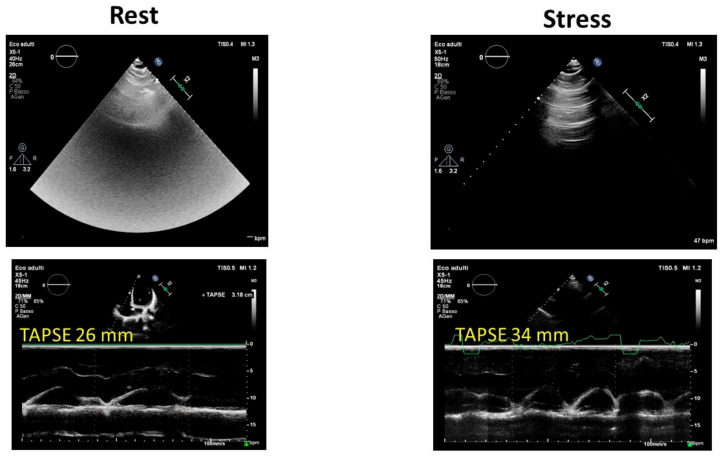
Exercise stress echocardiography in an endurance athlete: note the normal increase in tricuspid annular plane systolic excursion (TAPSE) and the mild increase in B-lines at peak effort. TAPSE: tricuspid annular plane systolic excursion.
